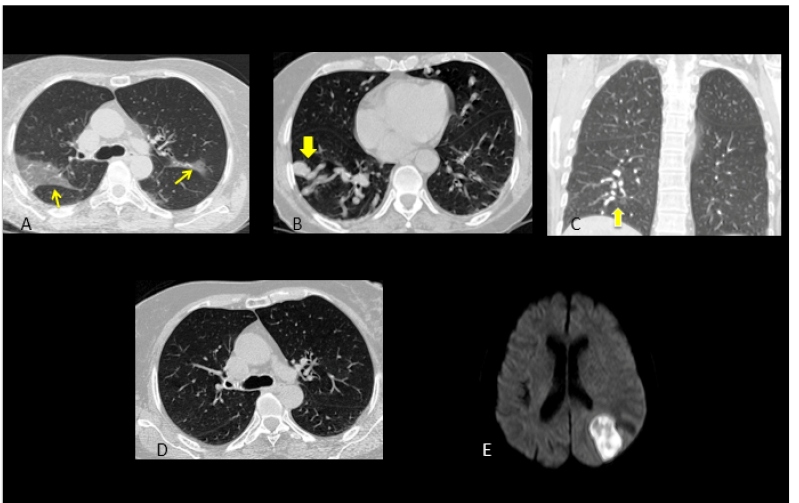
(A) Chest computed tomography, pulmonary window, axial view, showing bilateral multifocal areas of mainly peripheral consolidations (arrows). Chest computed tomography, pulmonary window, axial (B) and coronal (C) views, demonstrating multiple dilated and tortuous vessels consistent with pulmonary arteriovenous malformation (arrow). (D) Chest computed tomography, pulmonary window, axial view, performed 1 month earlier and showing no ground-glass opacities. (E) - Axial magnetic resonance imaging (diffusion-weighted imaging) showing restricted diffusion in the central content of a left parietal lesion, a finding consistent with pyogenic abscess.
